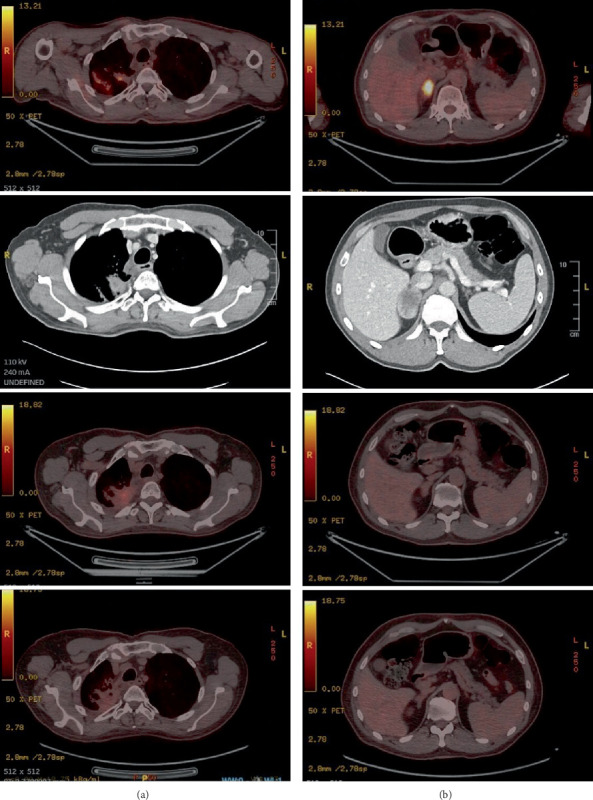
Sequential imaging demonstrating progression and therapeutic responses of the (a) primary lung lesion and the (b) adrenal metastasis across four time points. The top row (February 2024 PET-CT) shows initial progression with a newly detected adrenal metastasis. The second row (April 2024 CT) demonstrates further progression under pembrolizumab, prompting initiation of tepotinib. The third row (July 2024 PET-CT) reveals a complete metabolic response (CMR) in the adrenal lesion but oligoprogression of the lung lesion, leading to docetaxel addition. The bottom row (September 2024 PET-CT) confirms a partial metabolic response (PMR) of the lung lesion while the adrenal metastasis maintains CMR.
